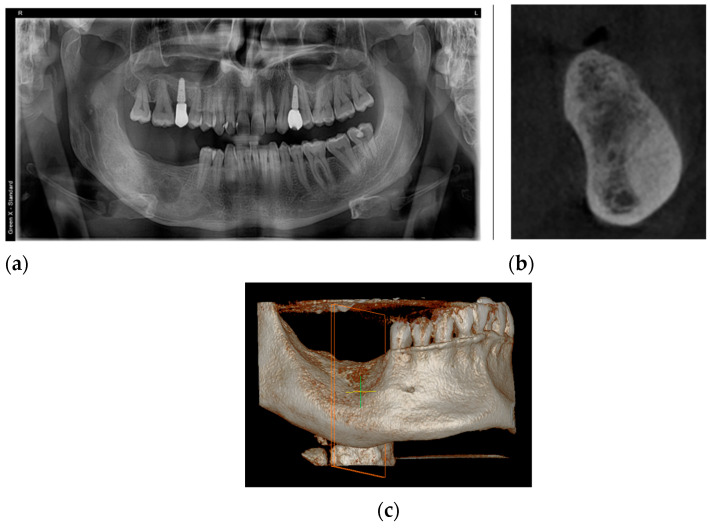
The aspect of the healing process, two years after recurrence surgery: (a) orthopantomography image; (b) cone-beam computerized tomography image; (c) the CBCT, 3D reconstruction view.
